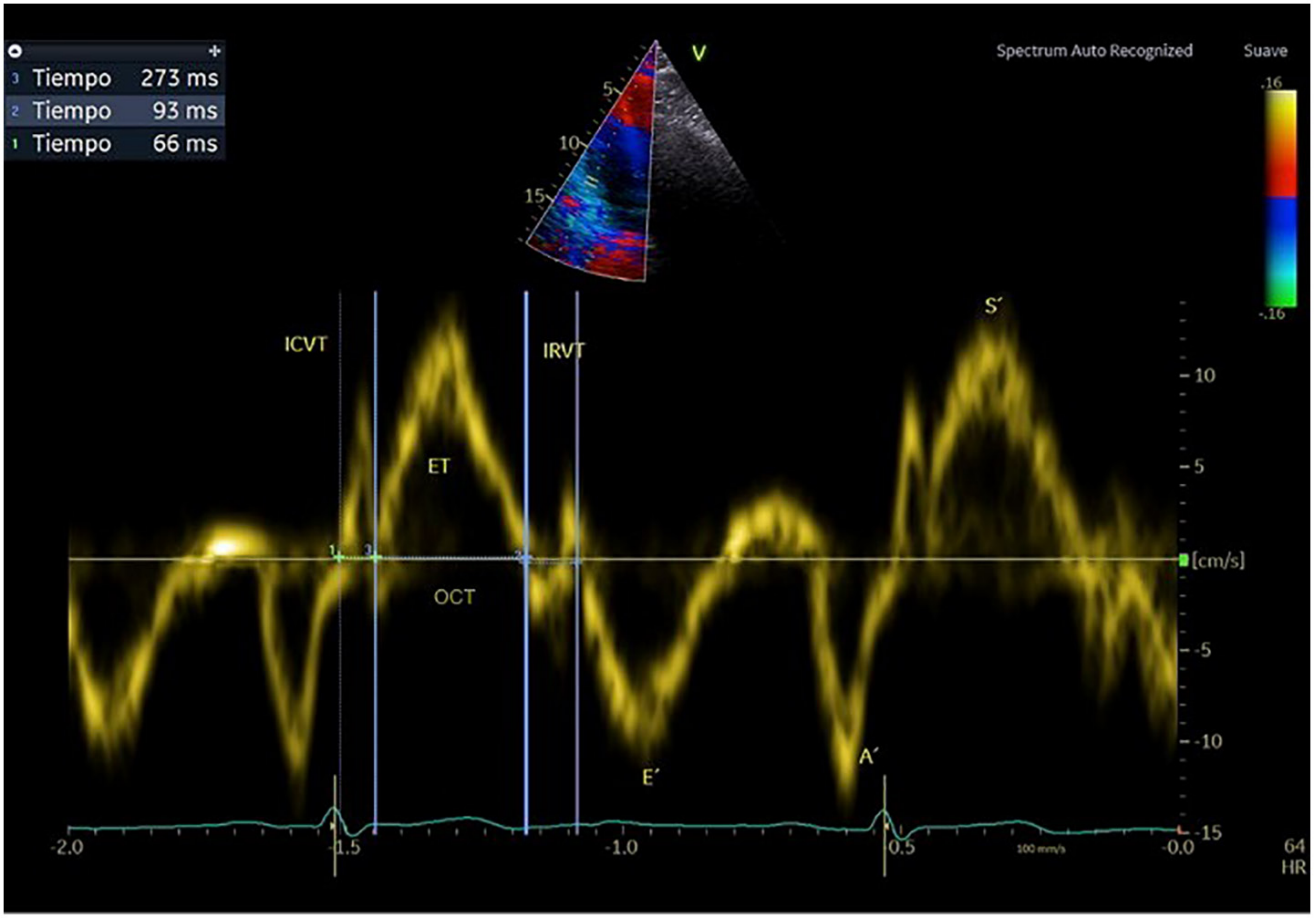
Tricuspid tissue Doppler velocity recording in apical 4-chamber view. E′: early diastolic wave; A′: late diastolic wave; S′: systolic wave. IVCT: isovolumetric contraction time; ET: ejection time; IVRT: isovolumetric relaxation time; OCT: opening and closing time
